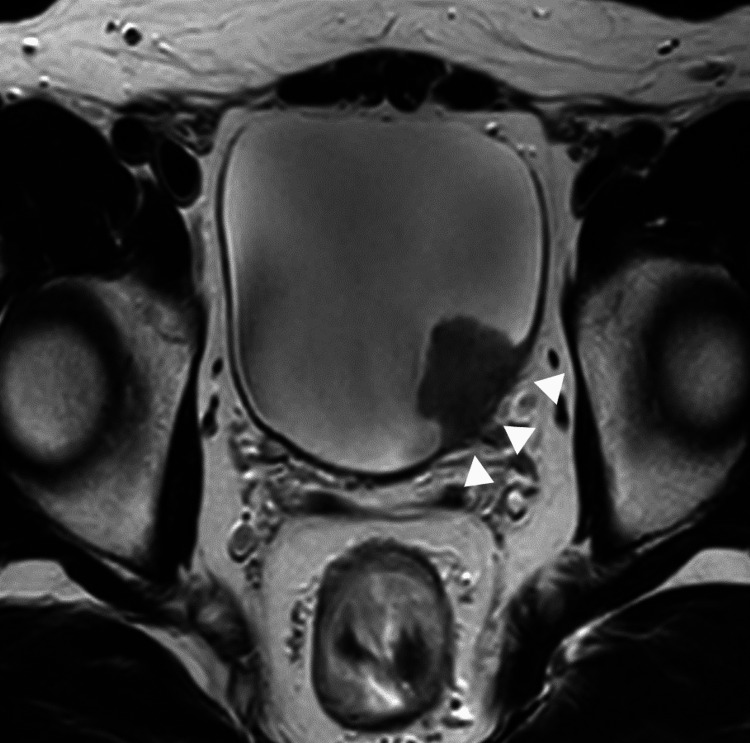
Bladder tumor before treatment. Magnetic resonance image displays multiple bladder tumors with invasion of the muscle layer (white arrowhead).
